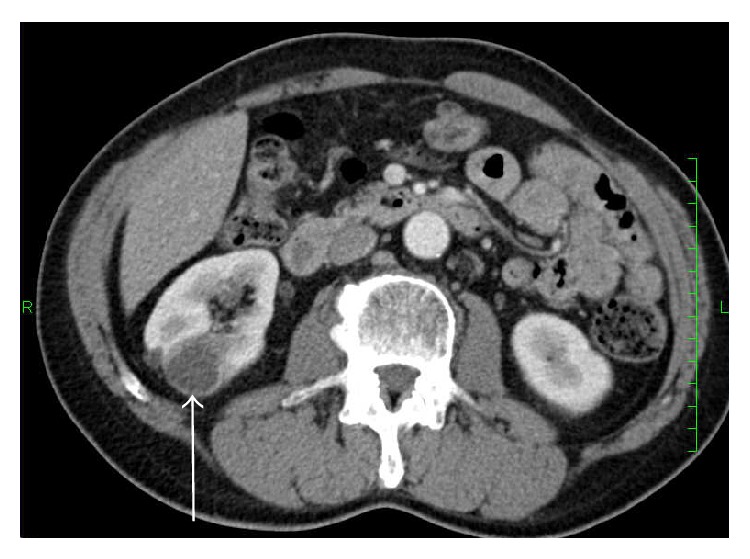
Computed tomography showing the isolated right renal mass (arrow).
